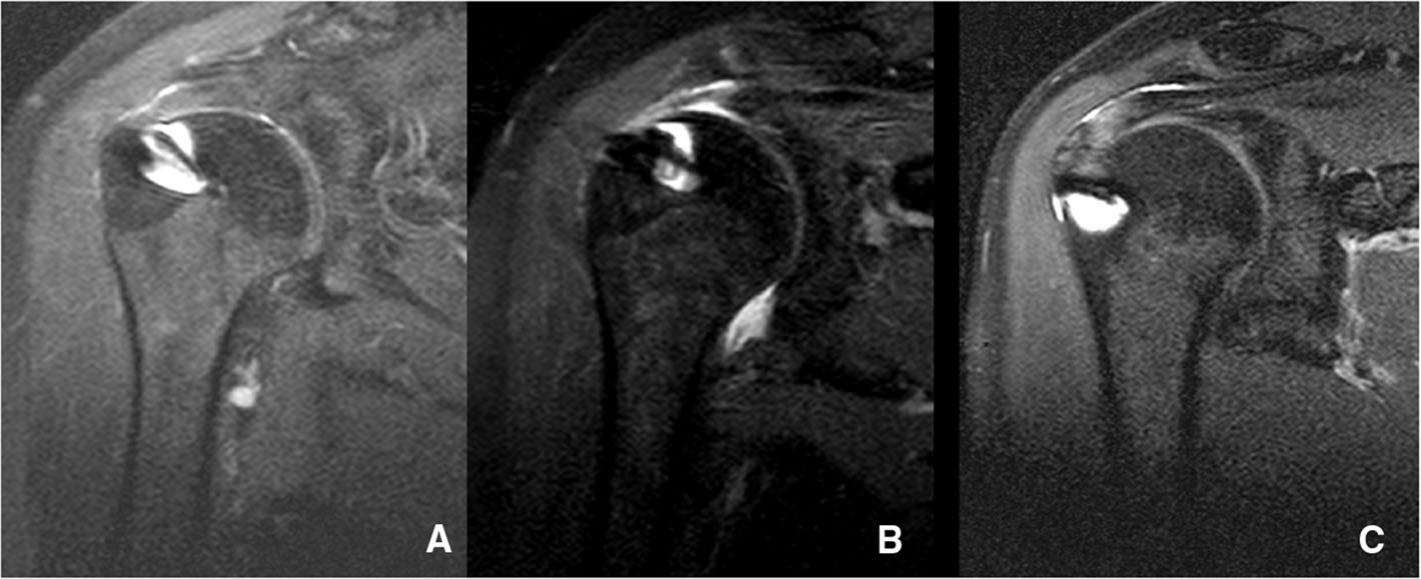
Assessment of repair integrity on T2-weighted MRI
